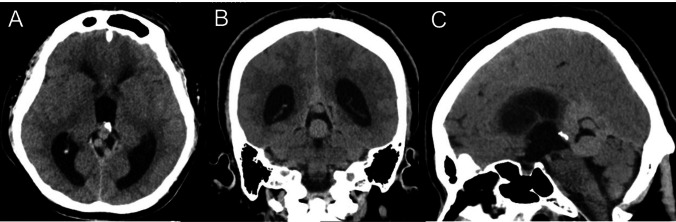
Initial head computed tomography (CT) of the patient, revealed several relatively high-density mass lesions in quadrigeminal cistern, resulting in occlusive hydrocephalus (A: axial view, B: coronal view, C: sagittal view)
